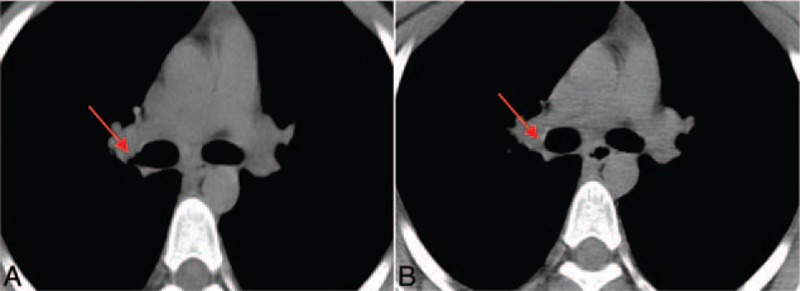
Findings of thoracic CT scanning. A: CT image before treatment. Local compression of the right bronchus caused stenosis of the bronchial lumen. In addition, shadows of intensity of soft tissues are also observed in the bronchus. B: CT image after treatment. The local compression of the right bronchus, as well as disappearance of stenosis was observed.
